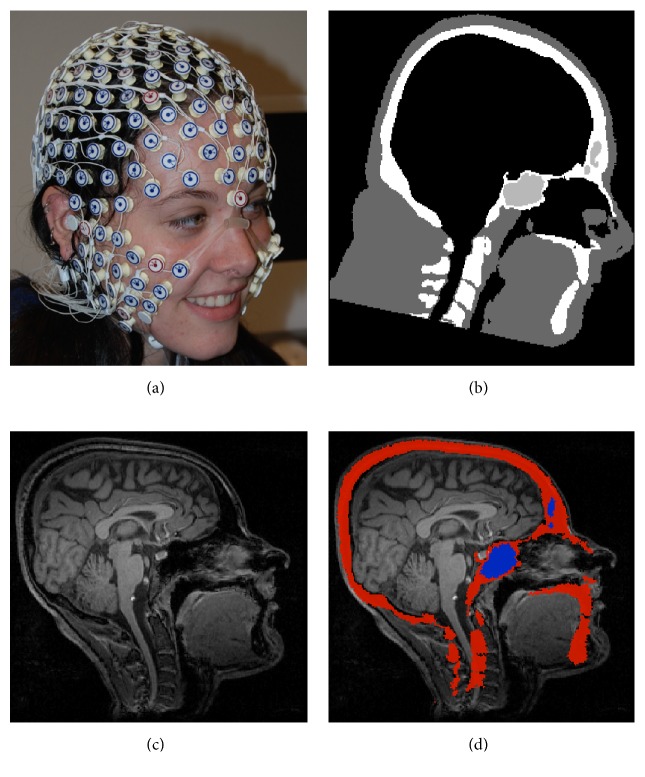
Skull Atlas-to-MRI registration with extensive warping of the skull to fit. (a) A volunteer in a 256 Net. (c) This person's MRI. (b) A database Atlas skull with a different shape. (d) Warp of the Atlas database skull to the volunteer's MRI.
